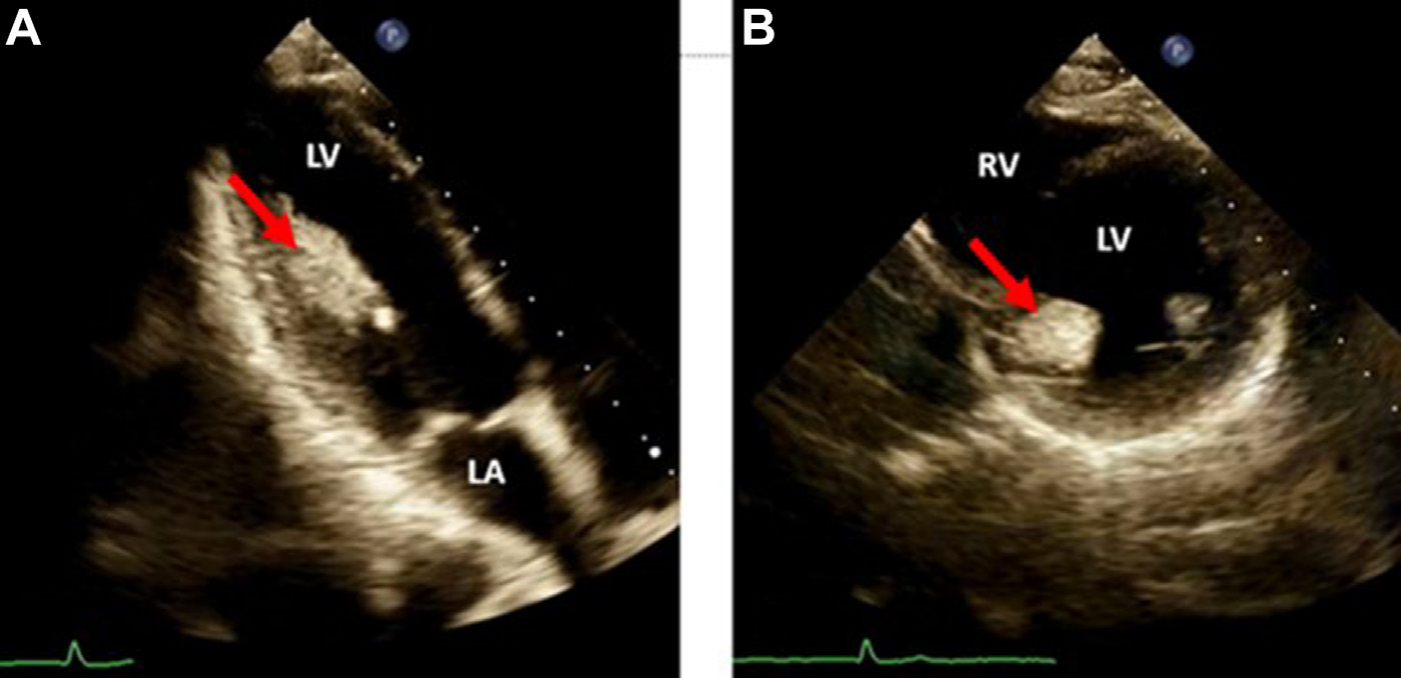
Two-dimensional TTE, rotated parasternal long-axis view, systolic phase (A) and parasternal short-axis diastolic phase (B), demonstrates a homogeneously hyperechoic mass *(arrows)* without echolucency involving the posteromedial papillary muscle; the anterolateral papillary muscle appears normal. *LA*, Left atrium; *LV*, left ventricle; *RV*, right ventricle.
